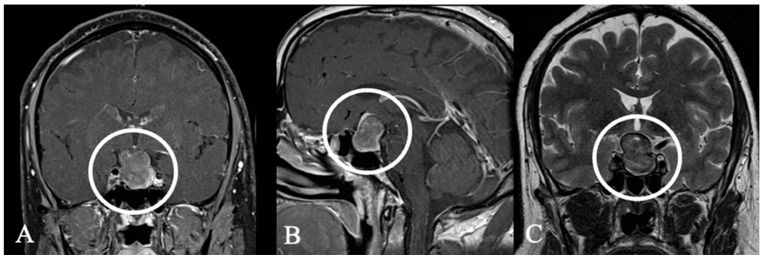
Necrotic cholesterol granuloma. A 51-year-old male patient presented with headaches and adynamia at initial diagnosis, along with reduced vision in the right eye. Endocrinological evaluation confirmed anterior pituitary insufficiency and arginine-vasopressin deficiency (AVD). (A,B) Coronal and sagittal contrast-enhanced MRI shows an inhomogeneous contrast-enhancing mass in the sella with low inhomogeneous signal in the T2 sequence (C). Radiologically, a hemorrhagic PitNET was diagnosed. Intraoperatively, the mass appeared as waxy, xanthochrome tissue. Complete resection was achieved.
